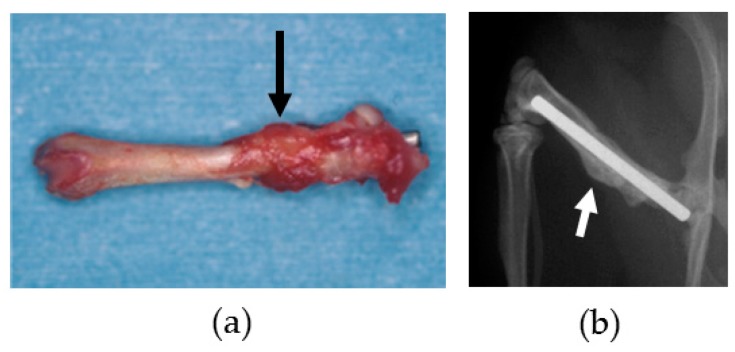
(a) Harvested femoral bone with BMG nail; (b) Radiograph of femoral bone and BMG nail at 12 weeks after osteotomy. The osteotomy sites were healed with callus formation (arrow).
